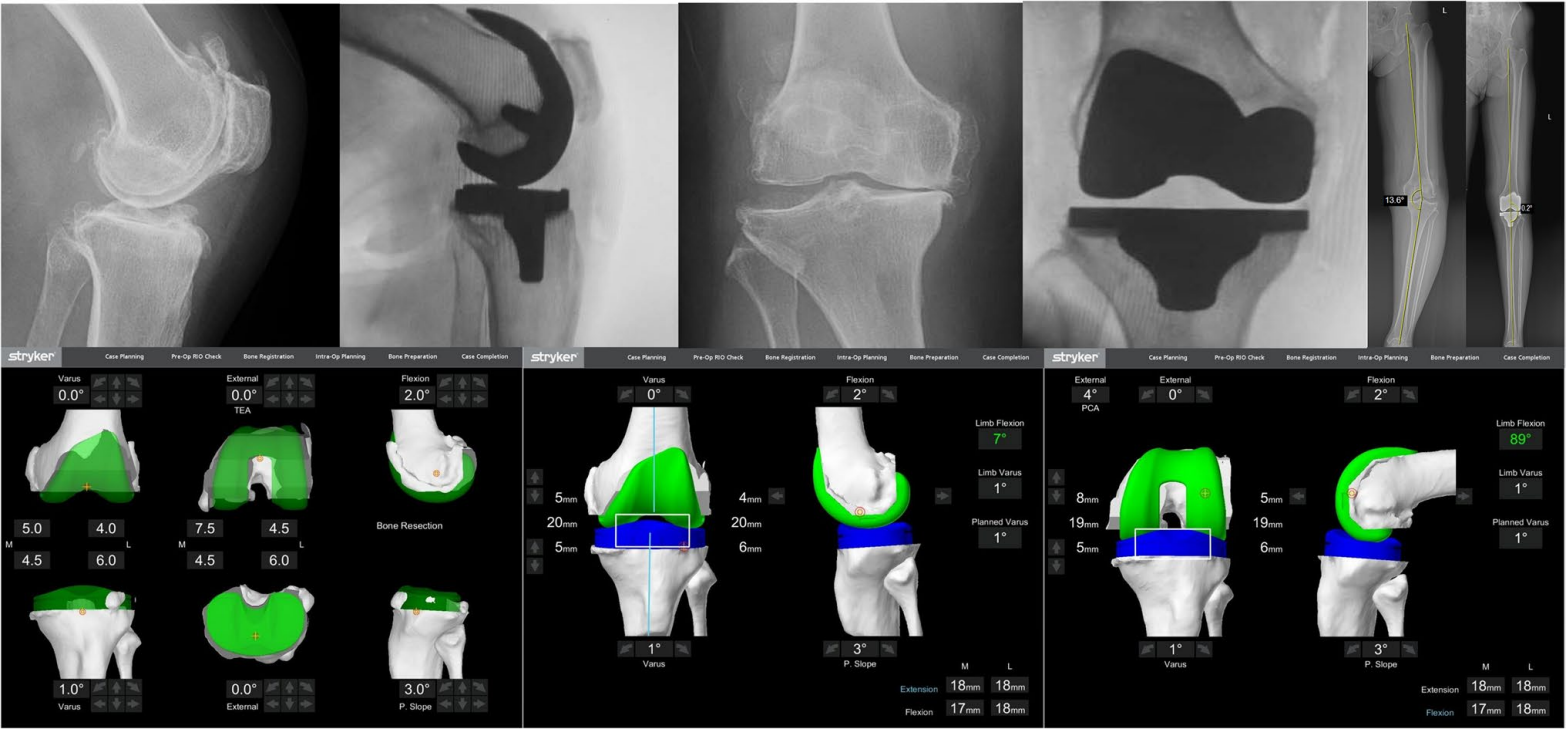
Typical Case: Female, 67 years old, left knee osteoarthritis, underwent MAKO robotic-assisted TKA surgery. Preoperative X-ray examination revealed narrowing of the medial tibiofemoral joint space, osteophyte formation, lip-like changes, and soft tissue swelling around the joint. The preoperative HKA is approximately 13.6°. Following surgery, the patient achieved balanced knee joint gaps in both extension and flexion states according to the preoperative osteotomy plan. Postoperative X-ray examination showed changes after left knee arthroplasty, with a well-maintained joint space and proper positioning of the prosthesis. The postoperative HKA is approximately 0.2°
